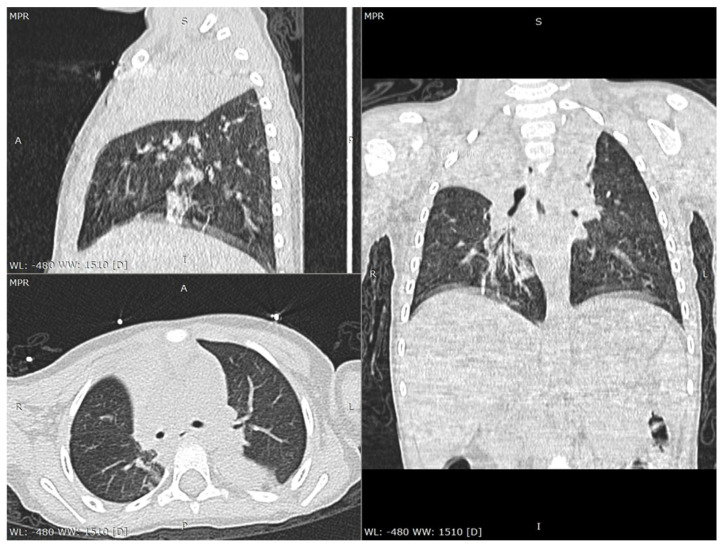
Chest CT scan lung window: multiplanar reconstructions (axial, sagittal and coronal views) show atelectatic consolidation of the right upper lobe.
